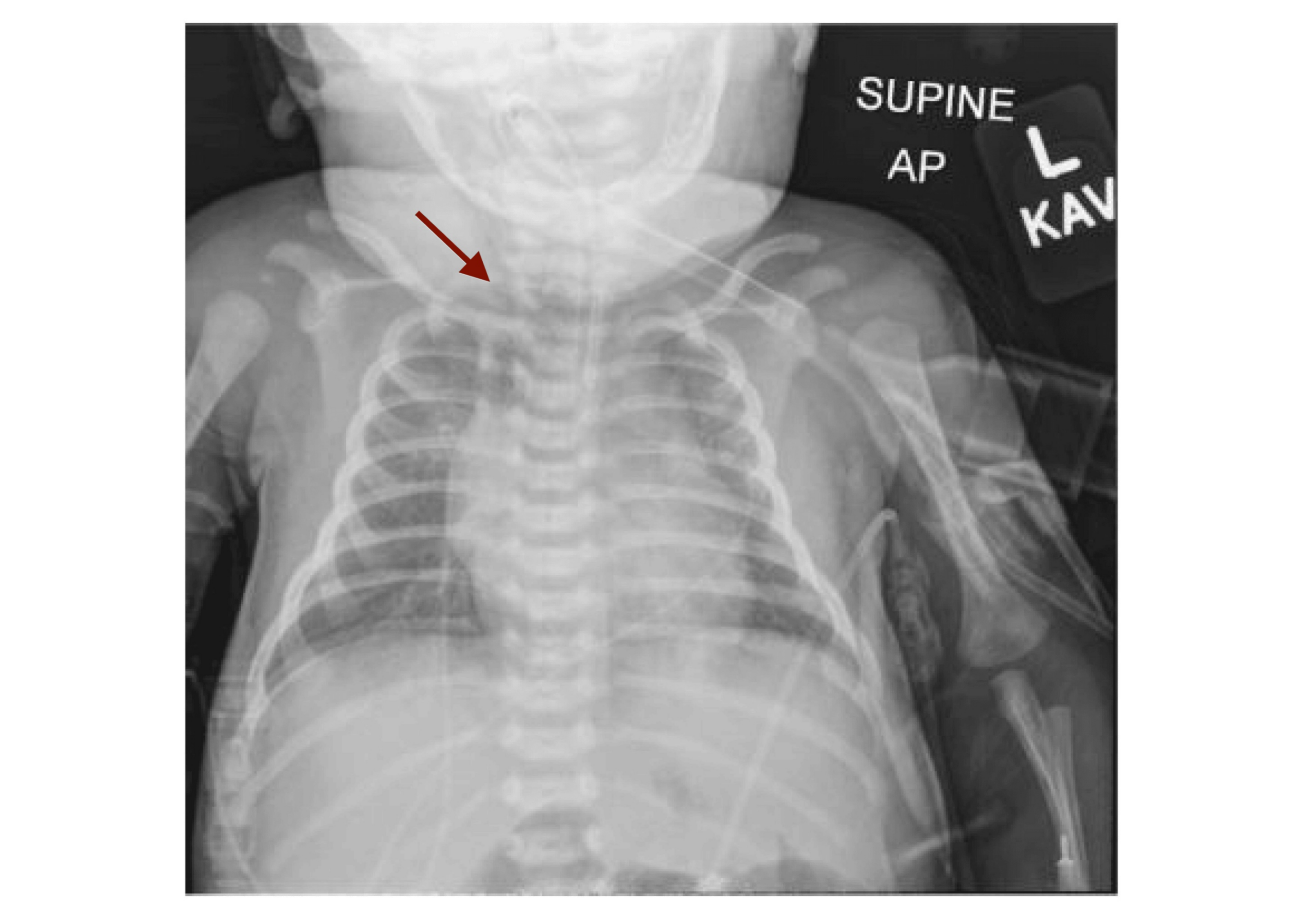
Anteroposterior chest radiograph showing pneumomediastinum (arrow) immediately following intubation.
